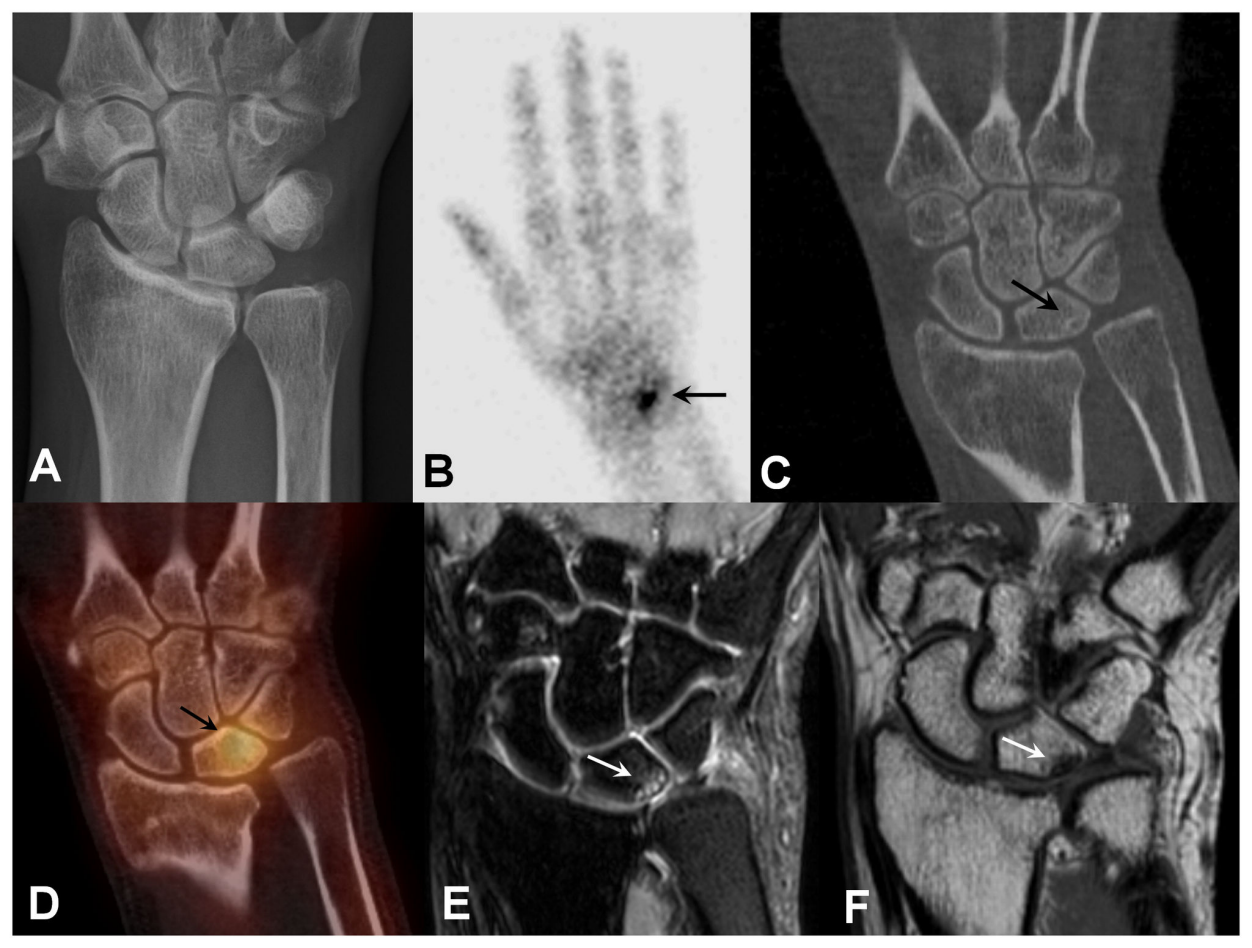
50-year-old female with ulnocarpal impaction syndrome. Relative overlength of the distal articular surface of the ulna on plain radiograph with localized geographic cystic-sclerotic changes in the medial proximal pole of the lunate and overprojection of TFCC calcification (a), focally increased radiotracer uptake in the carpal region on bone scan (b, arrow), subcortical cyst with rim sclerosis at the proximal ulnar pol of the lunate bone on CT (c, arrow), subcortical cyst displaying tracer accumulation on SPECT/CT fusion image (d, arrow), several subchondral cysts with adjacent alterations of bone marrow signal in the proximal ulnar pol of the lunate bone in PDw SPIR (e, arrow) and T1w image (f, arrow) on MRI.
